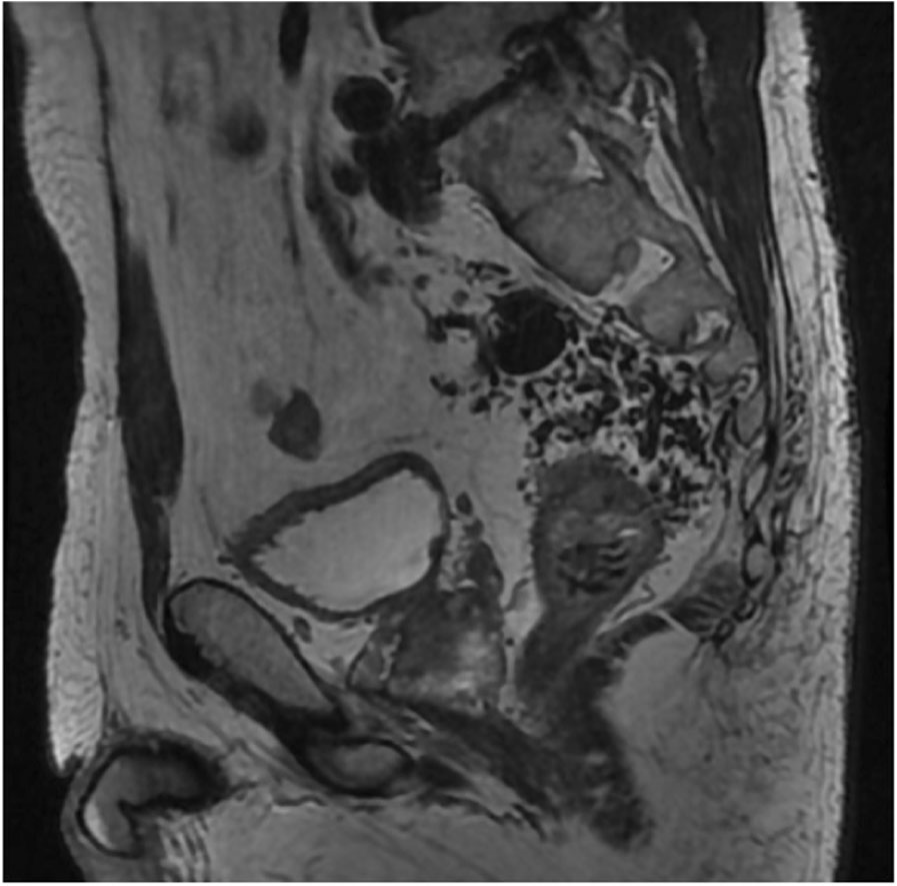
Abdominal enhanced magnetic resonance imaging revealed blood inflow from branches of the median sacral artery to APFs by flow void at the pelvic surface of the sacrum
